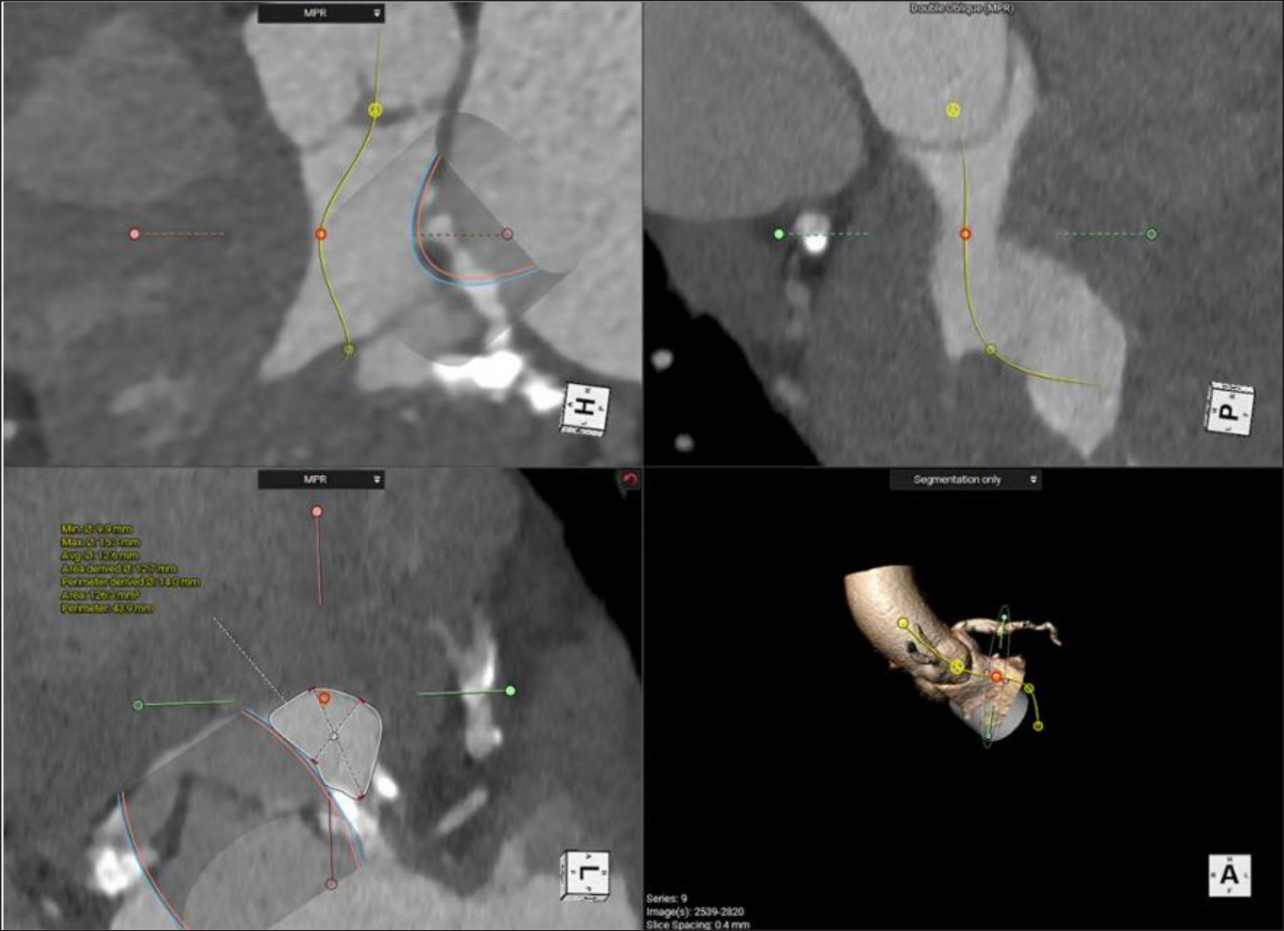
Cardiac computed tomography showing multiple views of the neo-left ventricular outflow tract (neo-LVOT) after implanting the virtual mitral valve. It measured 1.3 cm^2^, which is high risk for LVOT obstruction.
